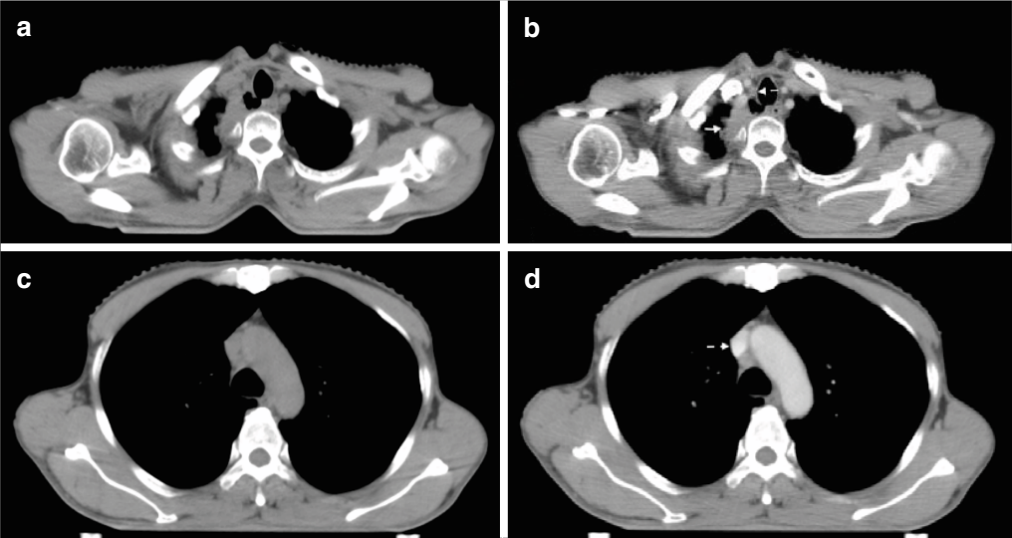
The Hounsfield units (HUs) of lesion in the plain 3D-CT (a) and CE-4D-CT (b) were 40 ± 6 and 62 ± 13, respectively; and the HUs of upper mediastinal lymph nodes in the plain 3DCT (c) and CE-4D-CT (d) were 33 ± 11 and 63 ± 16, respectively. The lesion (*solid arrow*) and nodes (*dotted arrow*) were clearly identified
